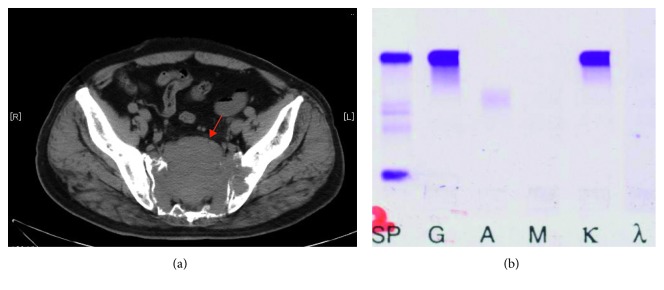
(a) Computed tomography (CT) at the time of multiple myeloma (MM) diagnosis. Sacral tumor and lytic bone involvement in the lumbar spine are shown (arrow). (b) Serum immunofixation at the time of MM diagnosis. SP, size marker; G IgG; A IgA; M IgM; κ, kappa chain; *λ,* lambda chain.
